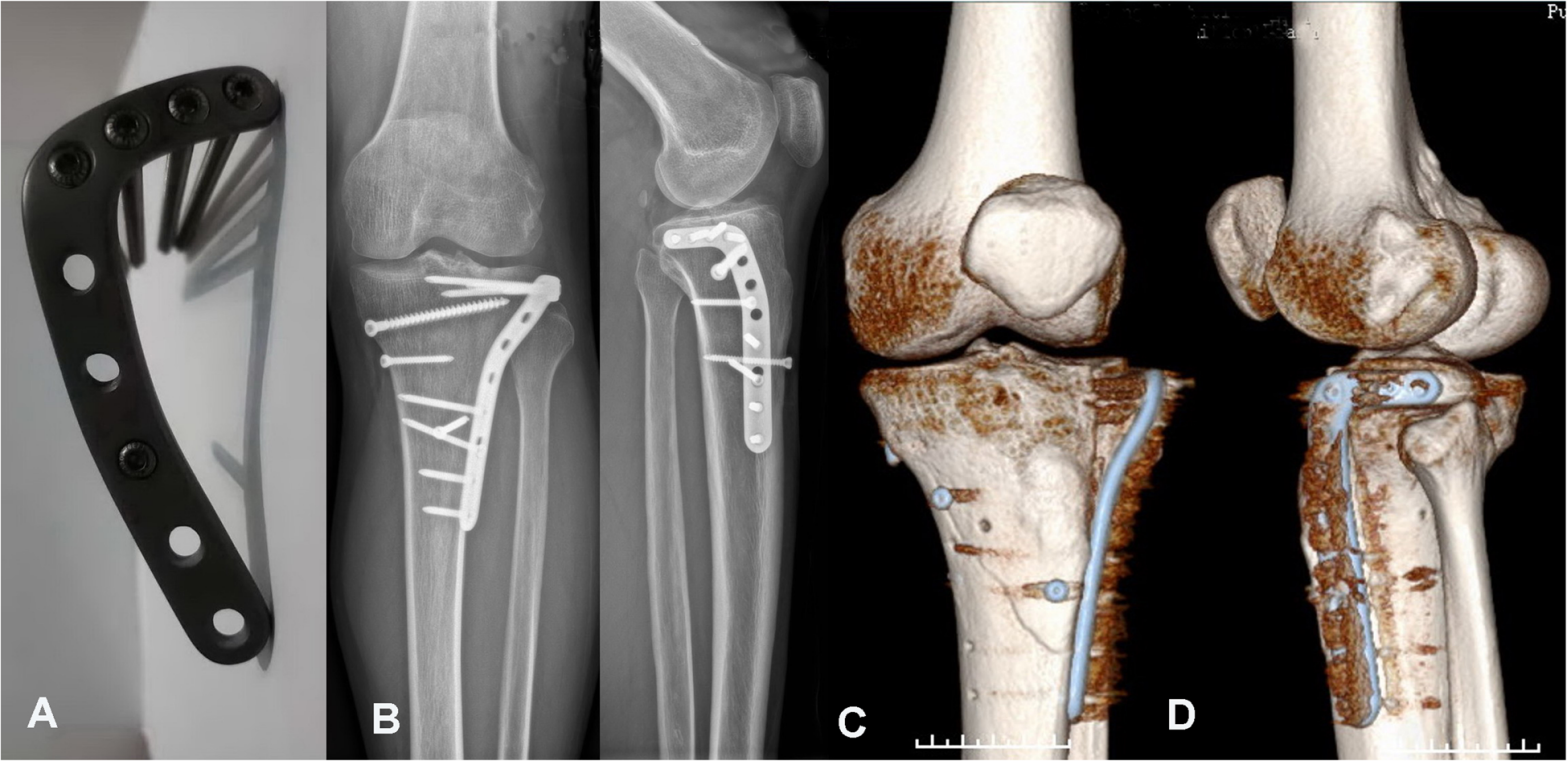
A. Our novel plate with a reverse L-shape placed through a modified anterolateral approach. B, C, and D. A patient with a Schatzker classification type V tibial plateau fracture fixed with our plate. B. Plain anteroposterior and lateral radiographs obtained post-operatively. C and D. Three-dimensional computed tomography (CT) reconstruction images obtained post-operatively showing the position of the plate
